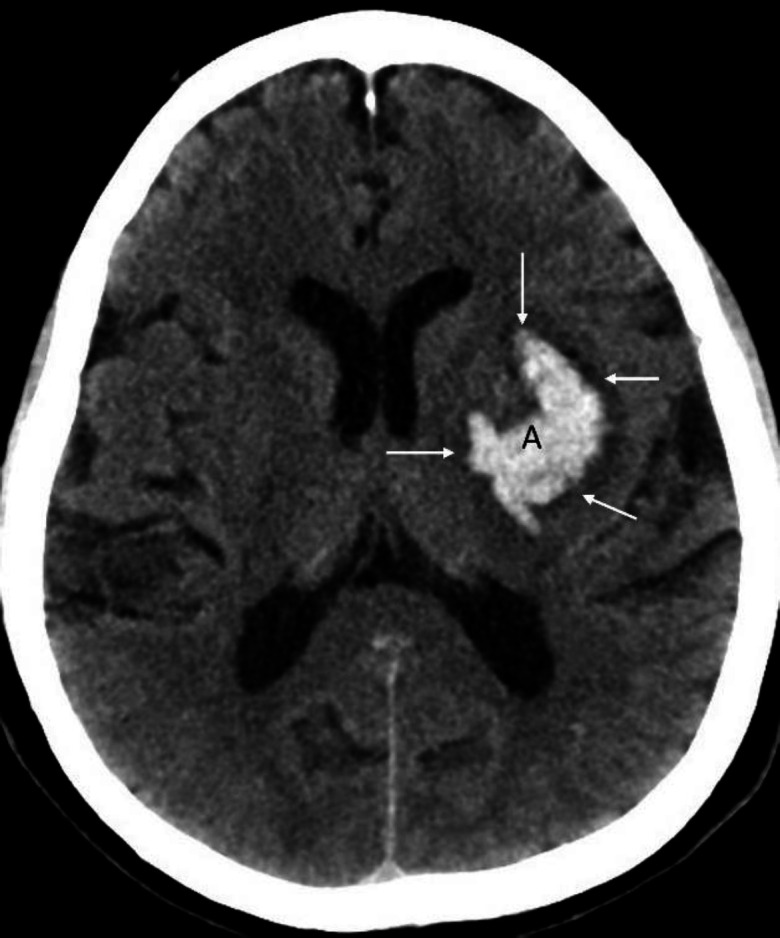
Non-contrast axial CT brain of a 79 years-old male with uncontrolled hypertension shows an intracerebral hemorrhage (A). Hemorrhage is centered over the left sided lentiform nucleus. Low density area surrounding the hemorrhage (arrows) represent peri-focal oedema. No significant mass effect on surrounding structures is noted on this image
